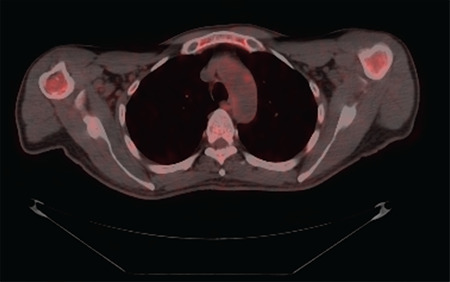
PET-CT showed aortic arch involvement and diffuse bone marrow FDG uptake. PET-CT: Positron emission tomography-computed tomography, FDG: fluorodeoxyglucose
